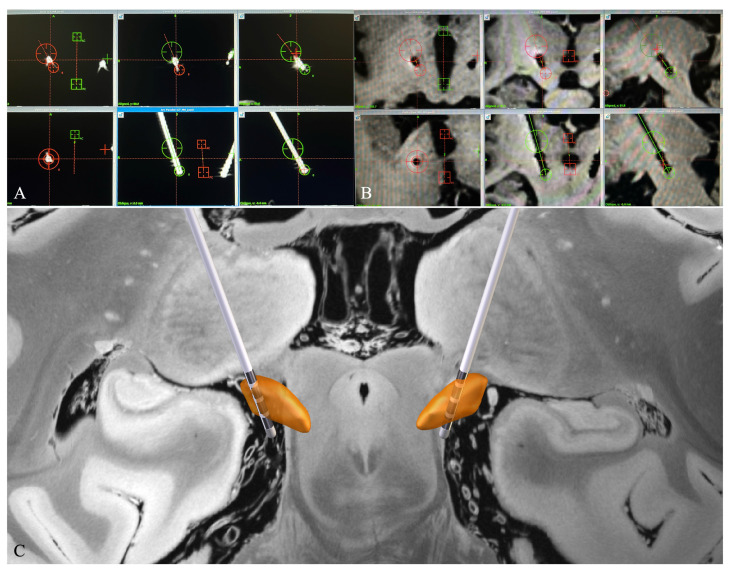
DBS electrode reconstruction using Surgiplan and Lead-DBS. Reconstruction of the DBS electrode in an example patient using Surgiplan with postoperative CT (A), Surgiplan with postoperative MRI (B), and Lead-DBS toolbox (C). In postoperative CT and MRI, the electrode is represented by a signal with a different intensity; in postoperative CT, the electrode is represented by a hyperintense electrode artifact (A), whereas in postoperative MRI, the electrode is estimated as an imaginary center of the hypointense signal (B).
